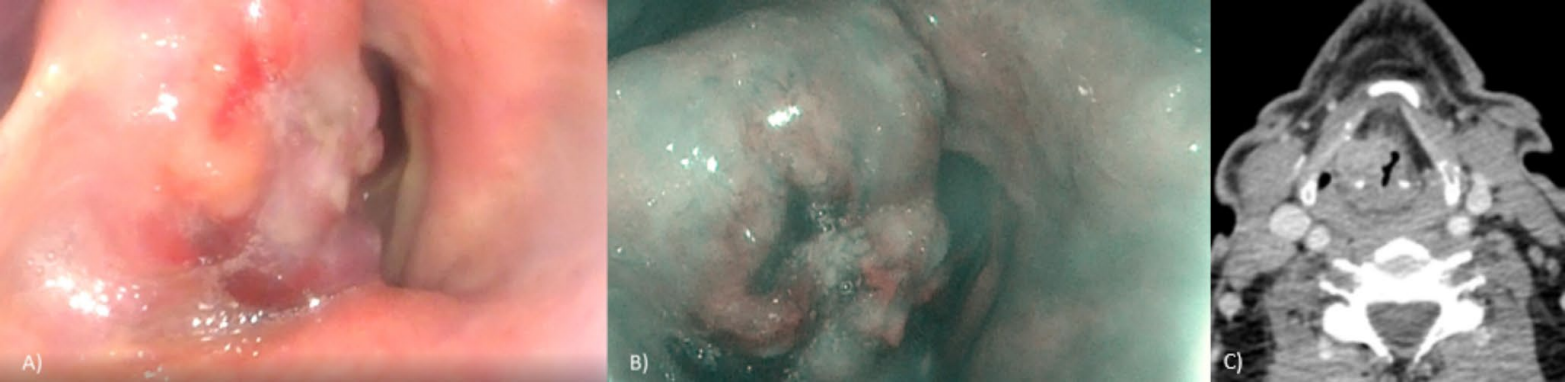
Videolaryngoscopy of the lesion in right hemilarynx (A); Narrow Band Imaging (NBI) of the lesion (B) CT scan of the lesion (axial projection) (C)
